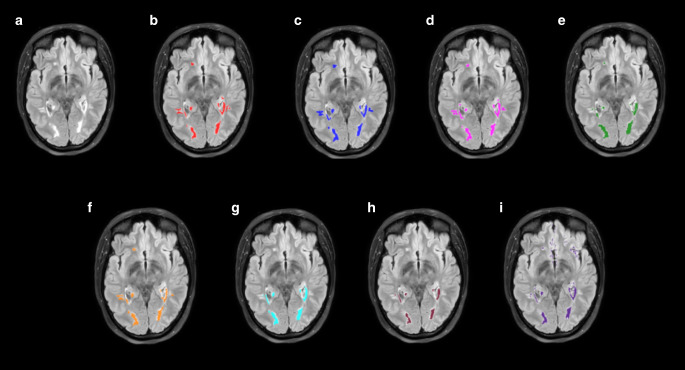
Example lesion delineations of all tested models including our implementation on an axial T2‑w FLAIR slice from a single patient. a T2‑w FLAIR, b expert 1, c adapted model (ours), d original model by Li et al., e nicMSlesions baseline, f nicMSlesions retrained, g LST-LGA, h LST_LPA, i BIANCA
